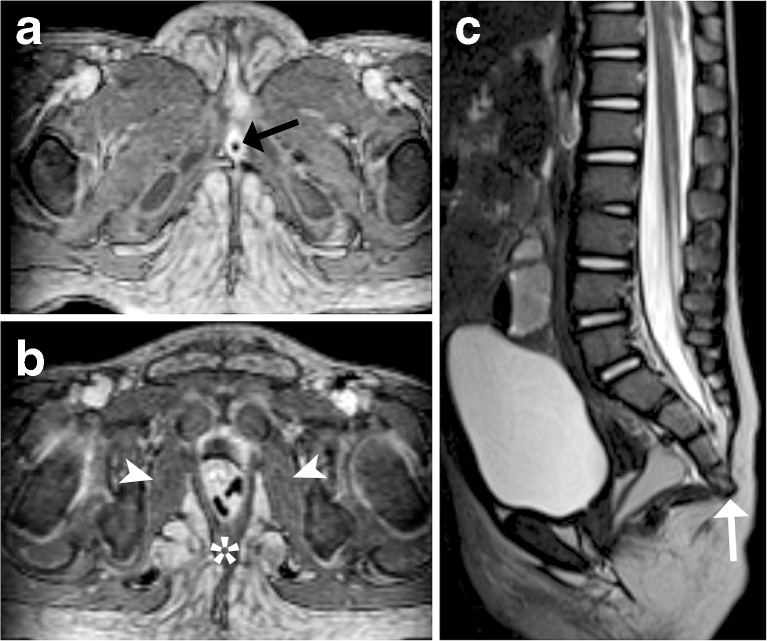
An MRI in a 1-year-old girl with cloacal malformation. (Axial T1-weighted turbo spin echo (a,b) for assessing the pelvic floor. A hypoplastic sacrum (*arrow*) is depicted in the sagittal steady-state free precession image (c)
